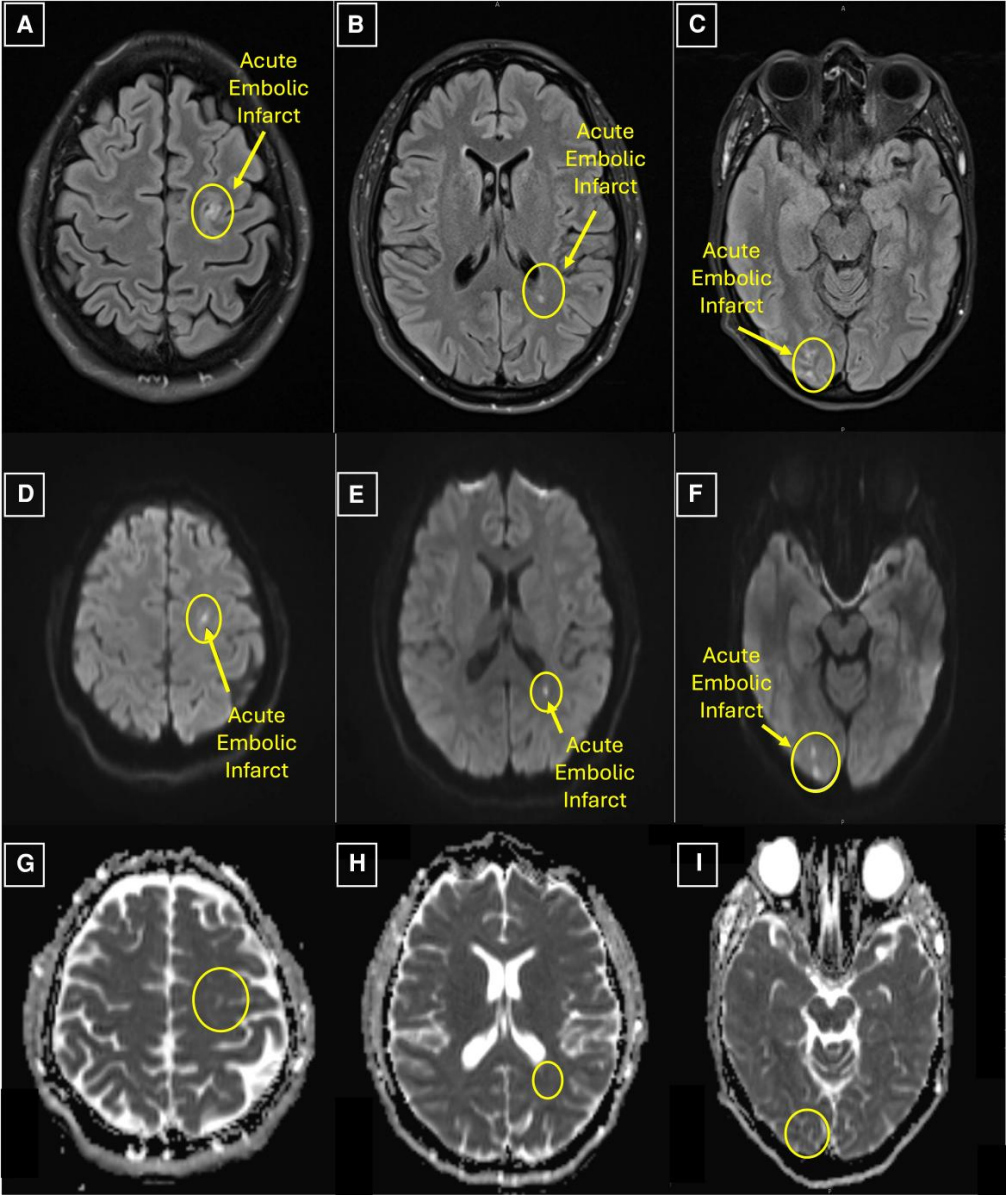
Case 2 (Septic Emboli): magnetic resonance imaging (MRI) in axial view with fluid attenuated inversion recovery (FLAIR) sequencing, diffusion-weighted imaging (DWI), and apparent diffusion coefficient (ADC) sequencing. There are multiple hyperintense foci seen on FLAIR imaging in both cerebral hemispheres at multiple levels throughout the brain (*Panels A, B, &C*). While hyperintensities on FLAIR sequencing are compatible with several diagnoses, they are considered a sign of early stroke. These hyperintense foci are visualized on DWI (*Panels D, E, &F*) but not ADC (*Panels G, H, & I*) sequencing; this pattern of hyperintense foci on FLAIR and DWI without a correlate on ADC is consistent with stroke. In the context of bacteraemia and endocarditis, this confirms a diagnosis of embolic infarcts from septic emboli.
